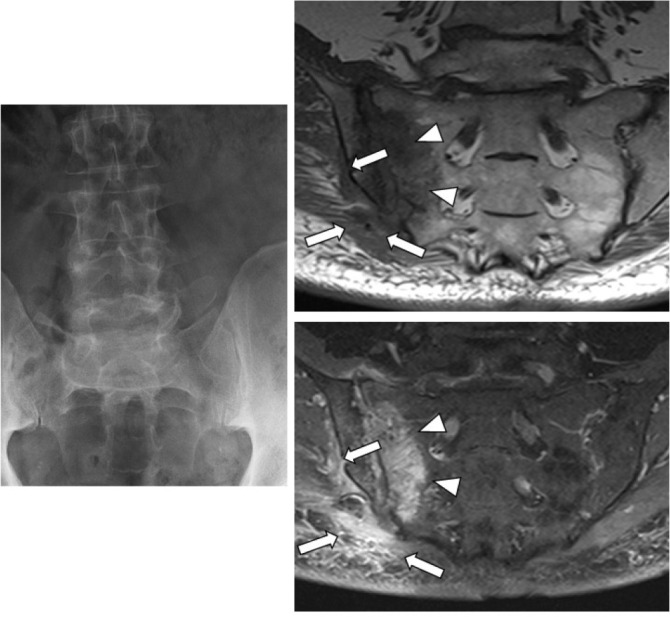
Radiograph and MRI of the SIJ of a 40 yo male patient with low back pain and elevated CRP. The radiograph shows no clear pathological findings. Oblique coronal MRI images after Gadolinium (Gd) application, showing hyperintense (inflammatory) signal periarticularly locates (arrowheads) but also in the gluteal muscle area (arrows), pointing towards a bacterial inflammation.
